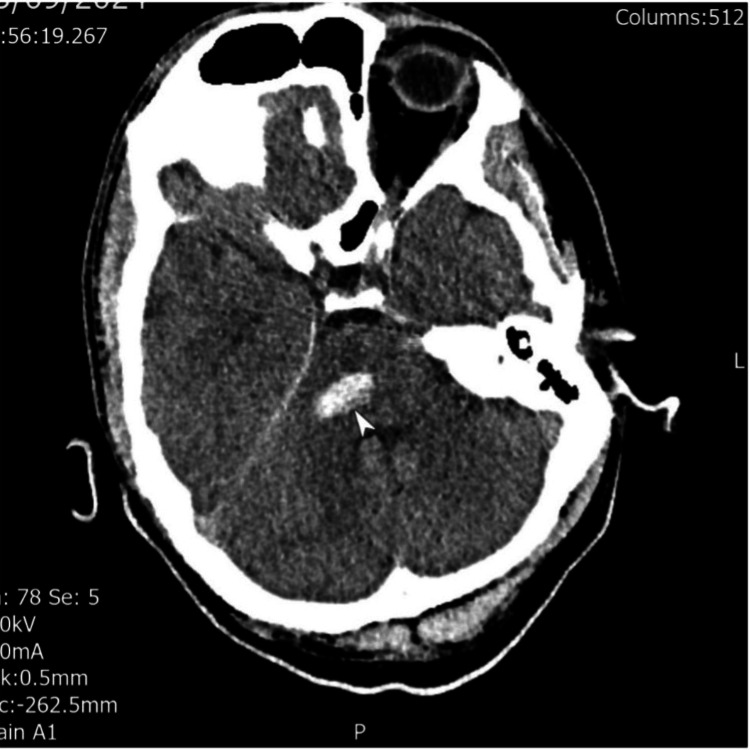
Admission CT brain-axial showing a right pontine bleed close to the middle cerebellar peduncle
